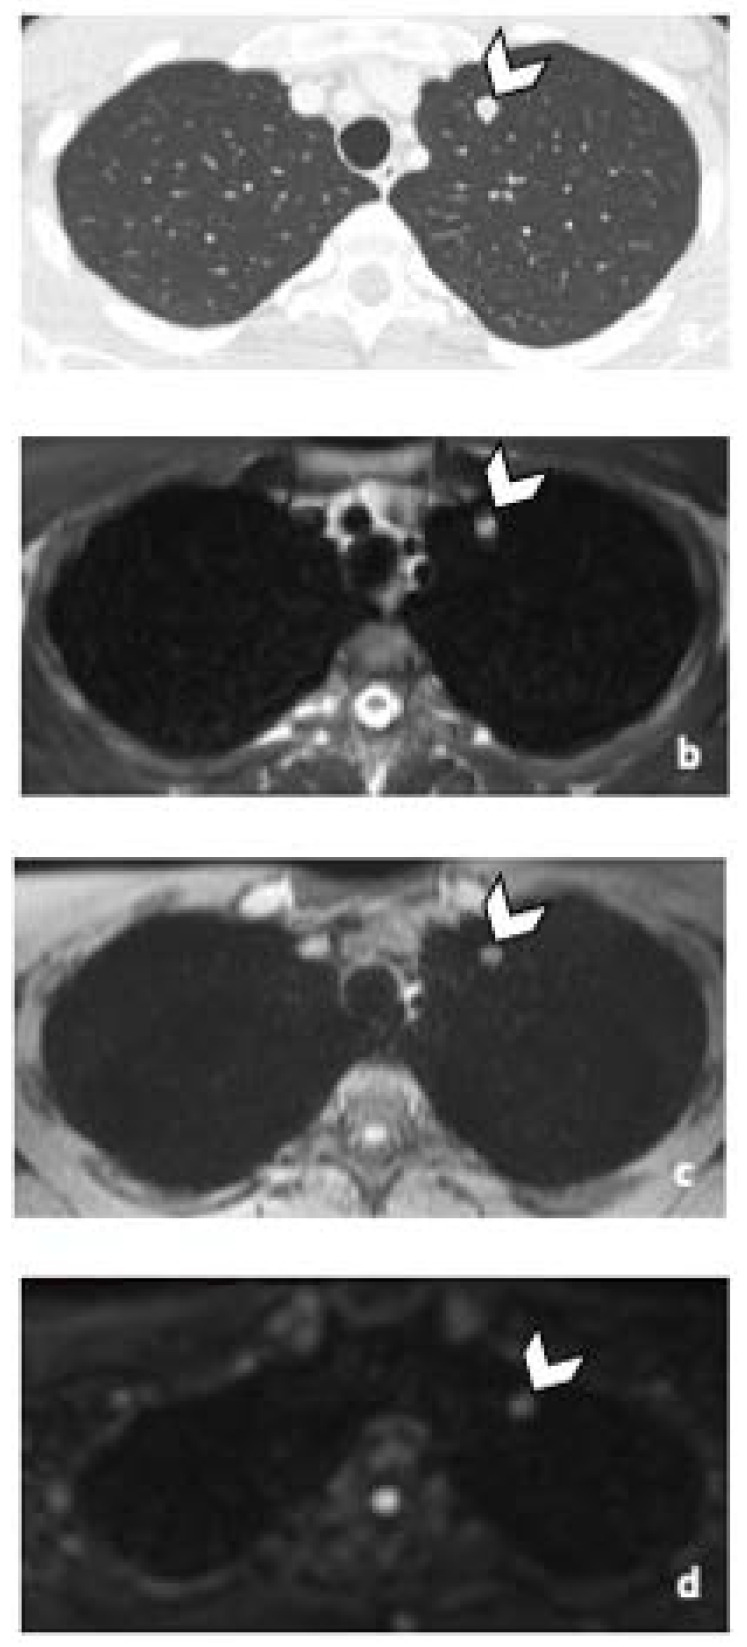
45-year-old man with seminoma. Axial CT image (a) and T2-weighted (b), 3D T1 GRE Fat-Sat (c) and high b-value DWI (b = 1000 s/mm^2^) (d) MR images. Left pulmonary nodule (arrowhead) detected by both readers during CT and MRI readings. Abbreviations: 3D: Three Dimensional, T1 GRE: T1 Gradient Echo, DWI: Diffusion Weighted Imaging, MRI: Magnetic Resonance Imaging, CT: Computed Tomography.
